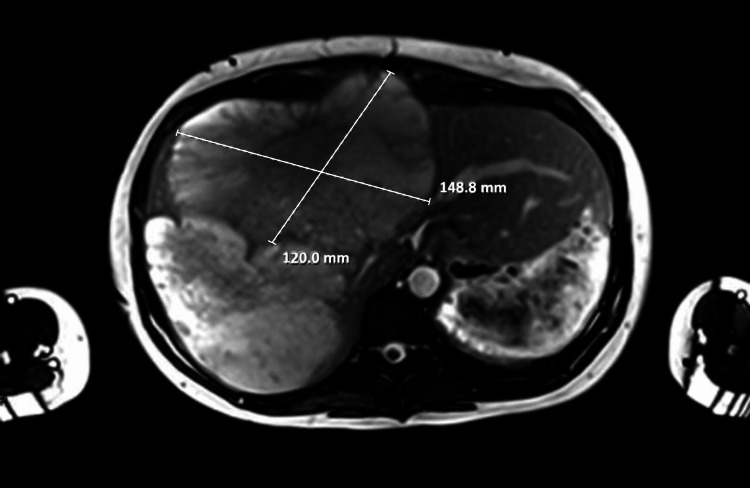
Sagittal MRI of the abdomen and pelvis showing 12 cm x 14.9 cm (120 mm x 148.8 mm) cystic liver mass alongside multiple small cystic masses MRI: magnetic resonance imaging
